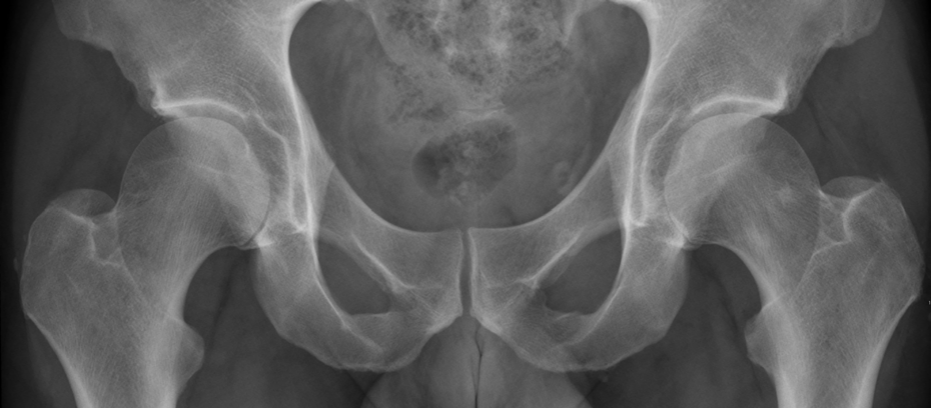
Pre-operative anteroposterior and lateral radiographs.
